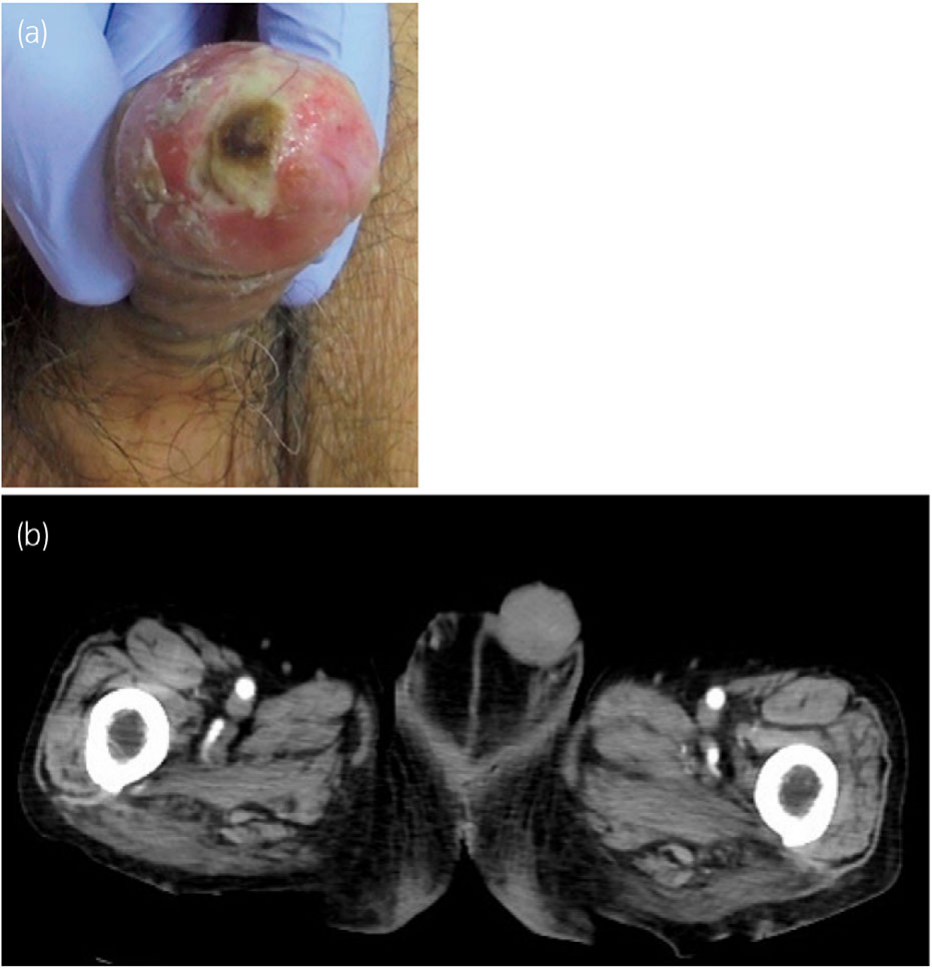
(a) A 10‐mm black ulcer is observed on the glans penis. (b) Contrast‐enhanced computed tomography image shows no evidence of penile ischemia.
